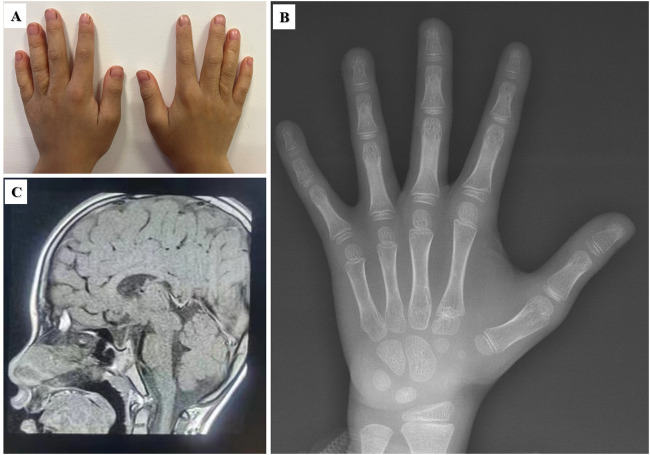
Clinical features of the eighth proband with the *NAA15* variant. (A) Surgical scar of partial syndactyly in both hands. (B) Bone age radiograph. CA: 9/11/12 years; BA: 7 years (C) Partial empty sella of the pituitary gland.
